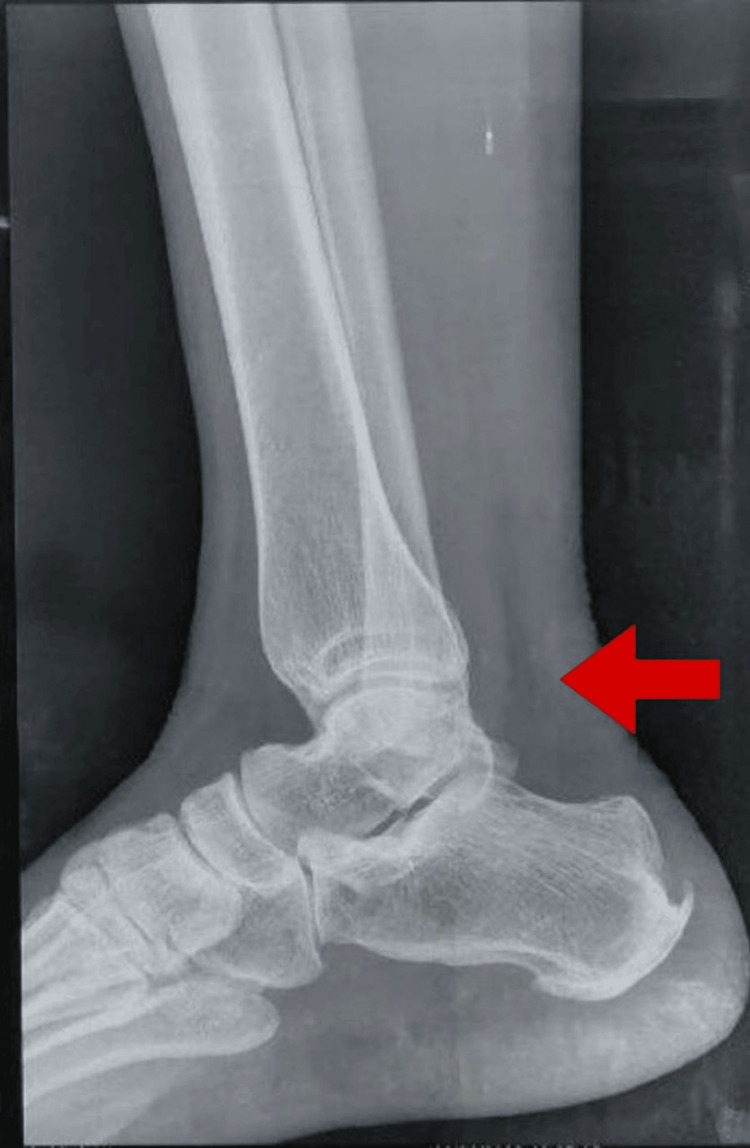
X-ray evidence of injury over the Achilles tendon (red arrow)
